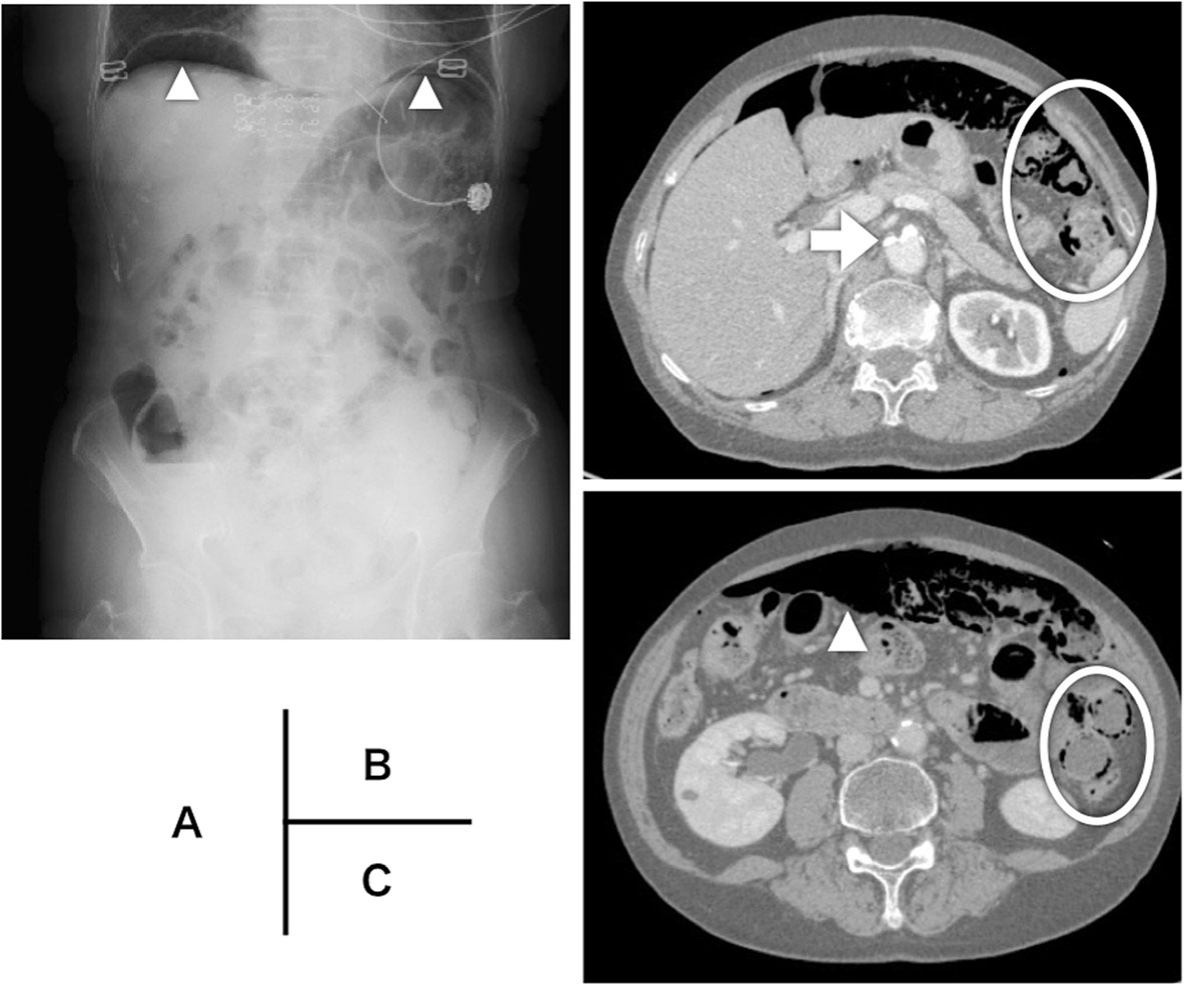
Abdominal plain radiography showing free air (a, triangle). Enhanced abdominal computed tomography showing severe calcification of the origin of the SMA (arrow), bubble-like intramural gas of the small bowel (circle), and pneumoperitoneum (triangle) (b, c)
